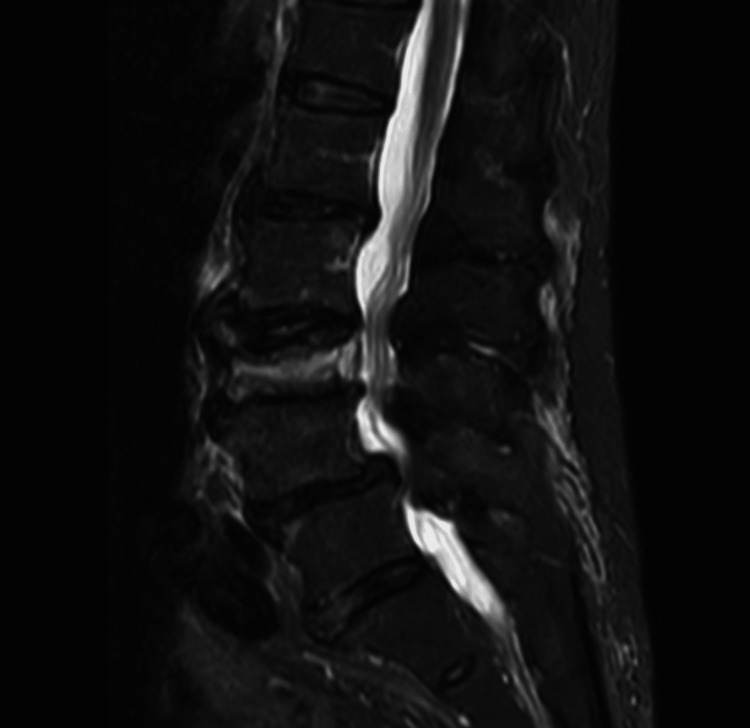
Sagittal view of lumbar MRI T2-STIR Magnetic resonance imaging (MRI T2-STIR) showed an L3 acute osteoporotic vertebral fracture (OVF). T2-STIR: T2-weighted short-tau inversion recovery
